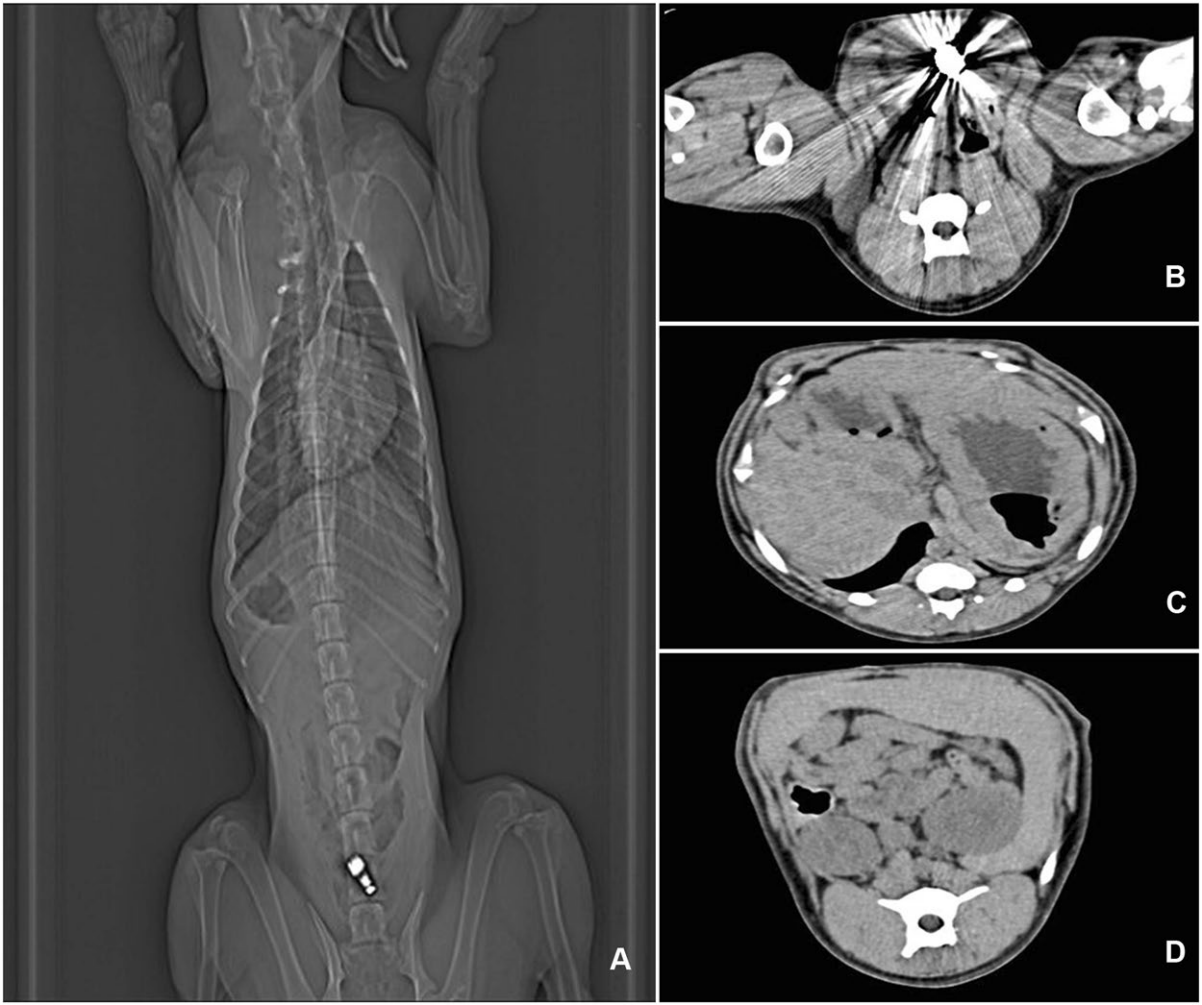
Radiographic examinations after administration of ultra-high frequency capsules. (A) Abdominal plain film after 2 hours’ vibration at ultra-high frequency. (B) CT image of stomach (arrow showing VC in the stomach). (C) CT image of intestine. (D) CT image of rectum.
